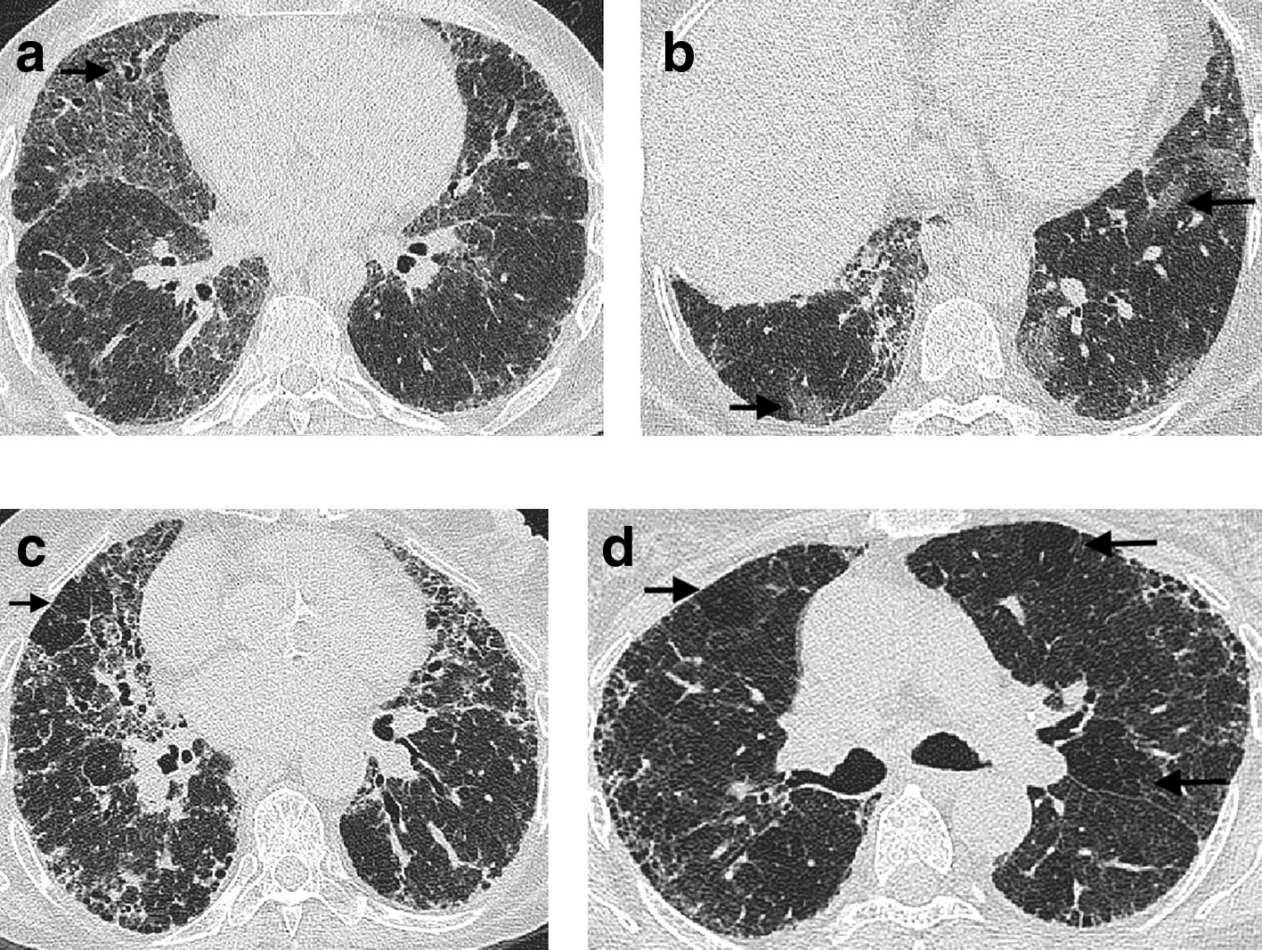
Axial CT image demonstrating ground glass density lying within areas of overlying reticulation and traction bronchiectasis (arrow) highly suggestive of fine fibrosis (a). When ground glass density lies separate to areas of fibrosis (arrow), it is far more likely to represent inflammation (b). Similarly, the low attenuation component of a mosaic attenuation pattern lying predominantly within fibrotic lung (arrow) is not specific for a diagnosis (c), but when it occurs in normal regions of lung (arrows), a diagnosis of chronic hypersensitivity pneumonitis can be made with greater confidence (d).
